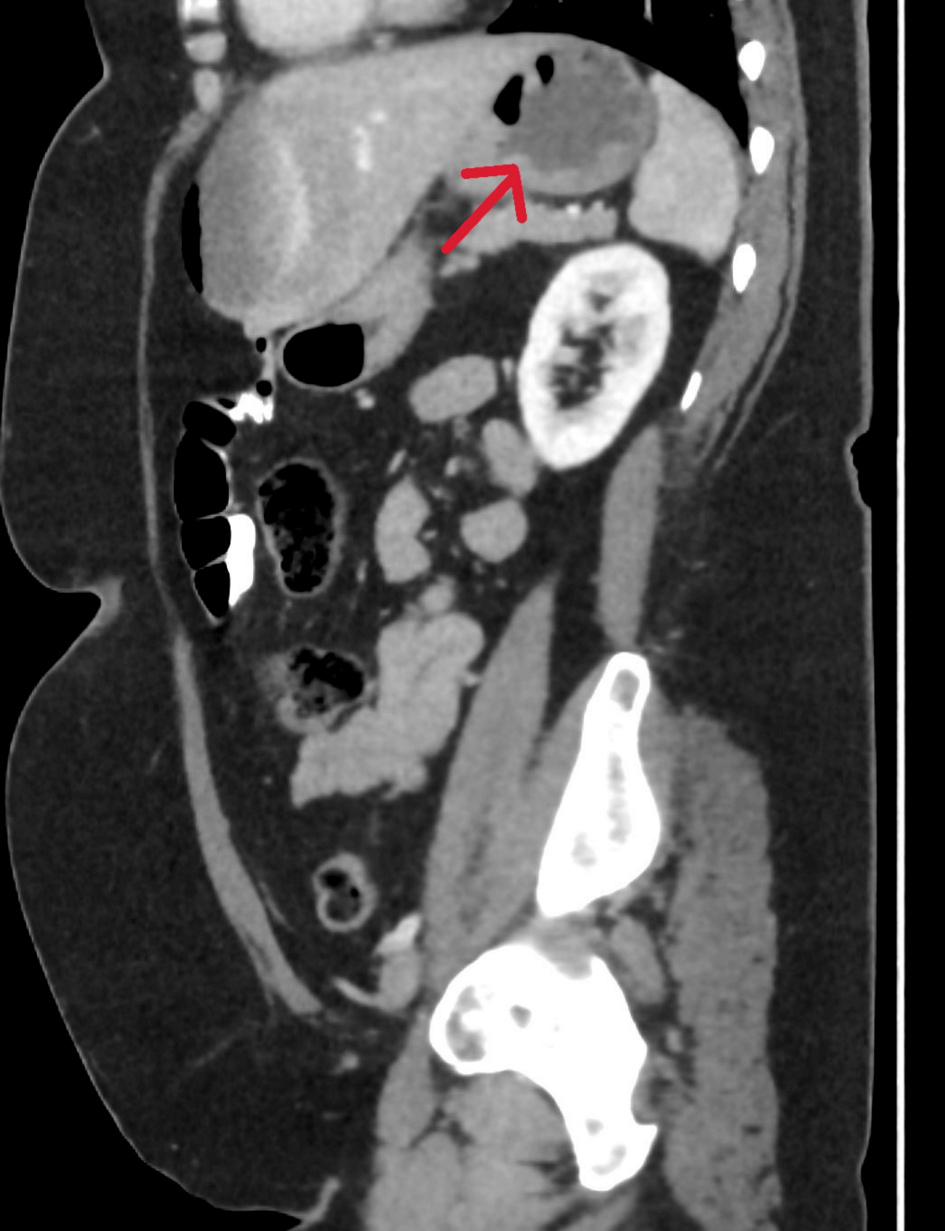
Sagittal CT scan revealing a well-defined perihepatic fluid collection consistent with a biloma
